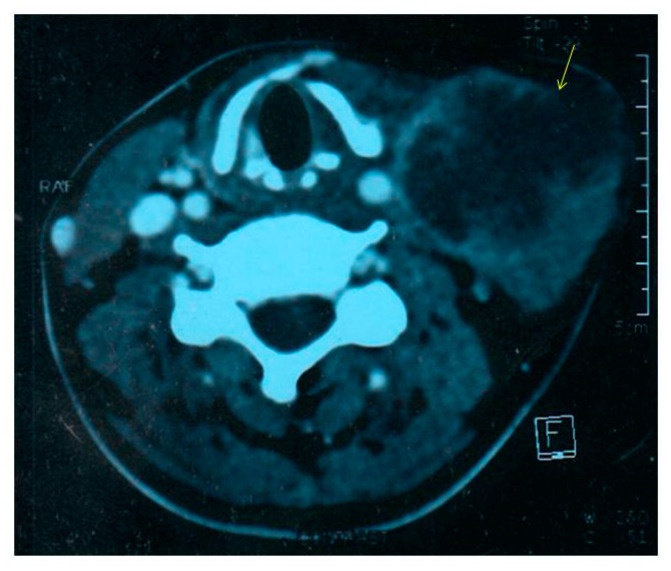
Contrast-enhanced CT neck showing left cervical abscess.
